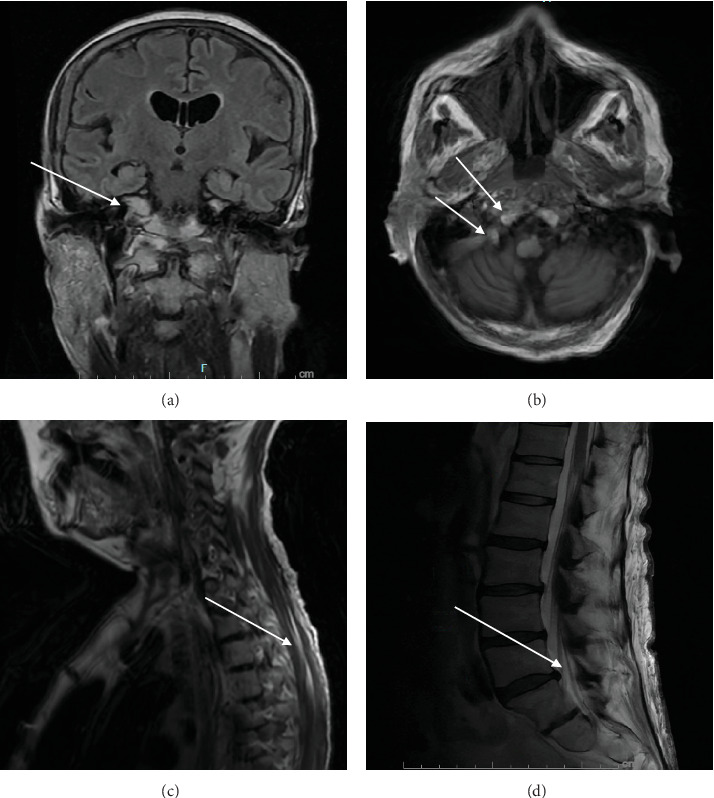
MRI brain: Subtle abnormal enhancement along the trigeminal nerves (a, arrow) and the seventh and eighth cranial nerves (b, arrows) at the internal auditory canal. MRI cervical spine: (c) partially visualized leptomeningeal enhancement of the upper cervical cord. MRI lumbar spine: (d) abnormal leptomeningeal enhancement and enhancing lumbar nerve roots. This is nonspecific and can be seen with infectious and inflammatory conditions including Guillain–Barré syndrome (GBS).
